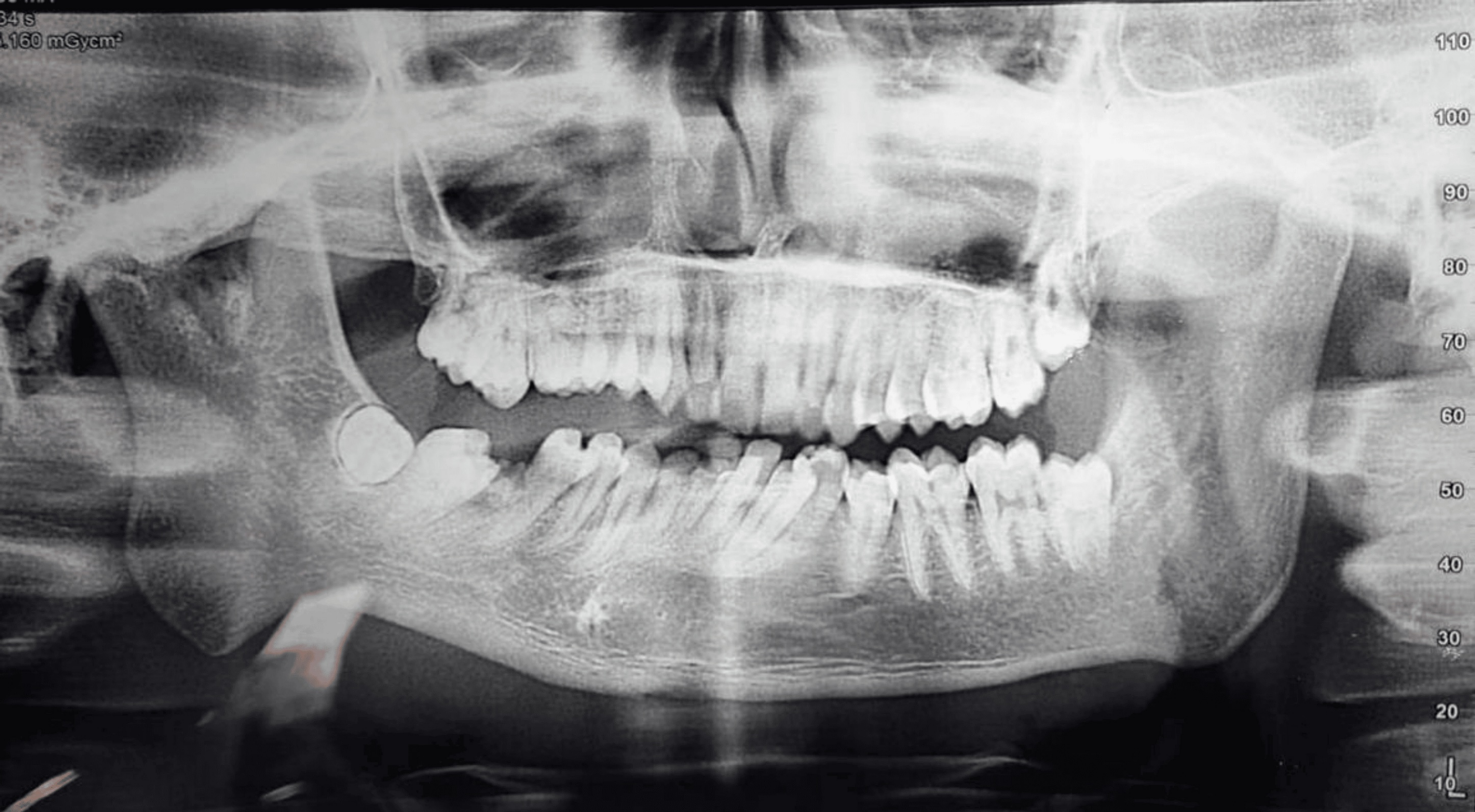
Preoperative orthopantomogram of the patient
